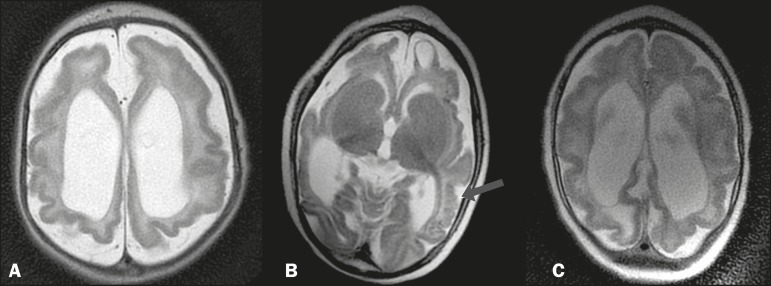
MRI, T2-weighted sequences in different patients, showing a pattern
